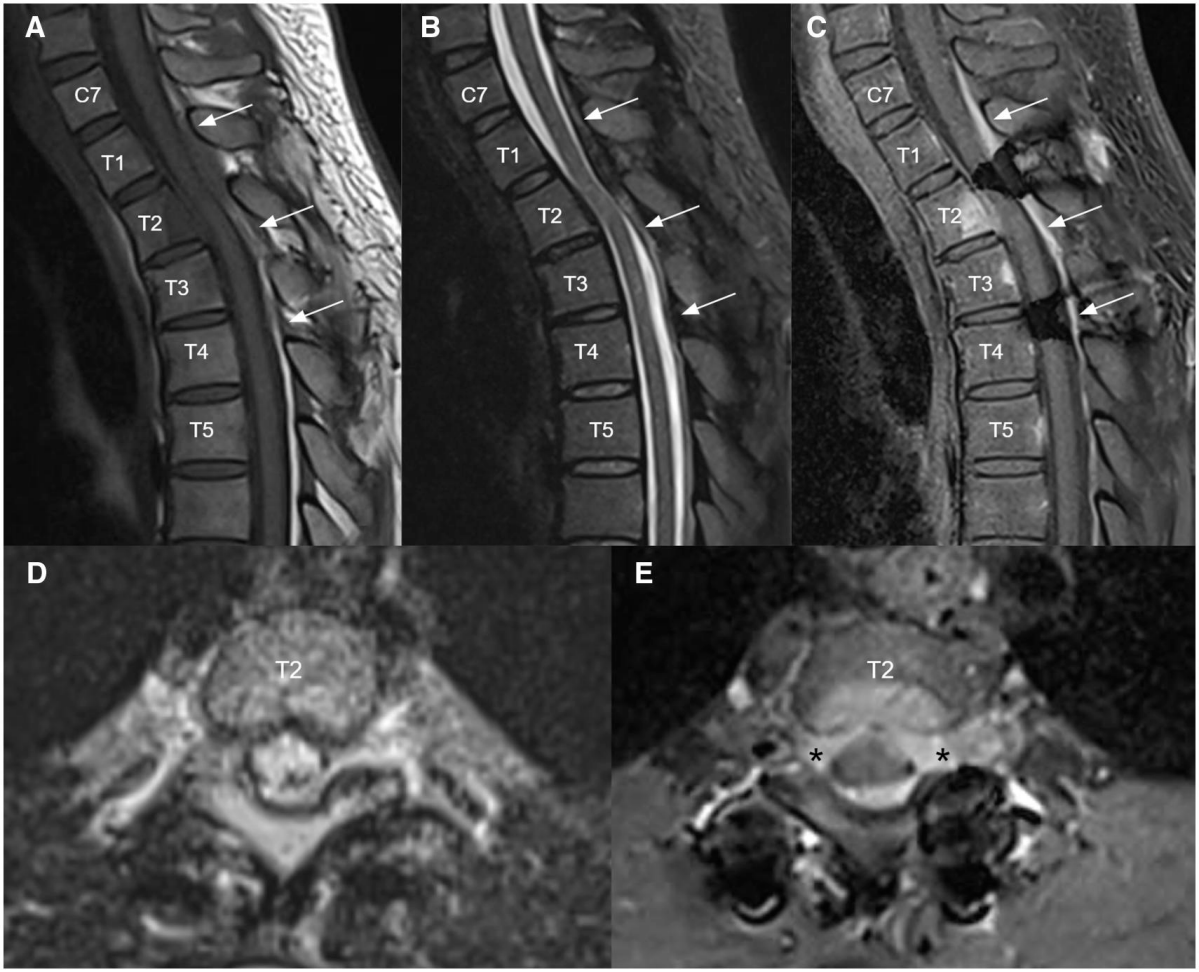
Follow up MRI 3 months after clinical recurrence upon low dose corticosteroid for a few years. Sagittal T1 weighted (A), T2 STIR (B), and T1 weighted post-contrast (C) MRI images of upper thoracic spine demonstrated a new enhancing infiltrative posterior epidural mass at C7 to T4 levels (white arrows) causing mild cord compression mainly at T2 level with cord edema. Axial T2 stir (D) and T1 weighted post-contrast (E) at T2 level showed that the epidural mass infiltrates into bilateral T2 lateral recesses and bilateral T2/3 intervertebral foramina (black asterisks). A new enhancing lesion was also noted at the posterior aspect of T2 vertebral body with overlying thin anterior epidural thickening (C, E). Mild residue T2 STIR hyperintense signal intensity (B) in the spinal cord at T3 level was compatible with old insult.
